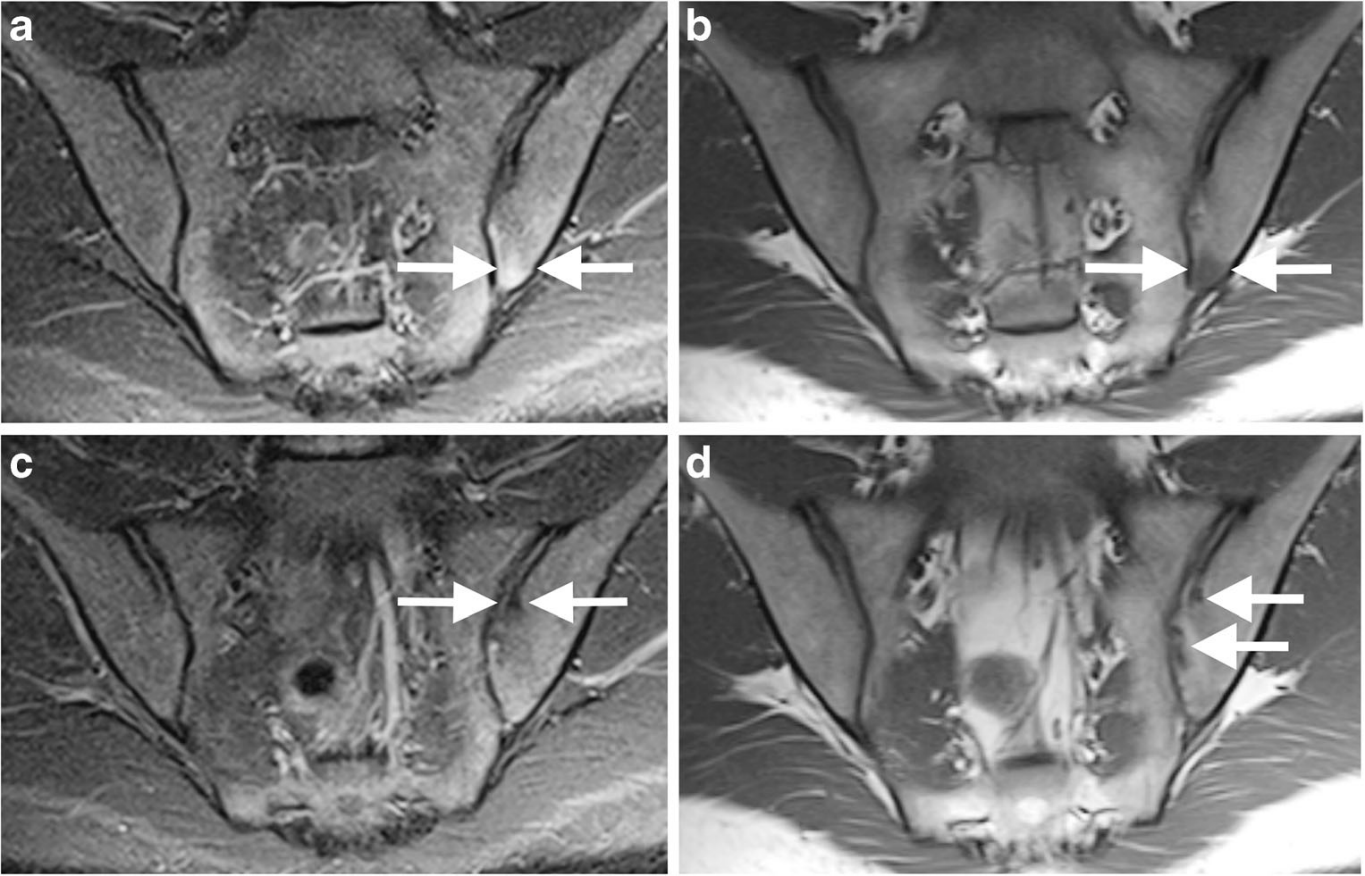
A 15-year-old girl with reactive arthritis and clinical sacroiliitis. a-b Coronal oblique short tau inversion recovery (STIR) spin echo (TR 4,000 ms, TE 36 ms) (a) and T1-weighted spin echo (TR 679 ms, TE 12 ms) (b) images demonstrate oedema on the iliac side of the inferior left sacroiliac joint (*arrows*), seen as high signal in (a) STIR and low signal in (b). c-d Coronal oblique STIR spin echo (c) and T1-weighted spin echo (d) images at a different location show broad-based erosions (*arrows*) more superiorly on the iliac side of the left sacroiliac joint, better appreciated in (d)
